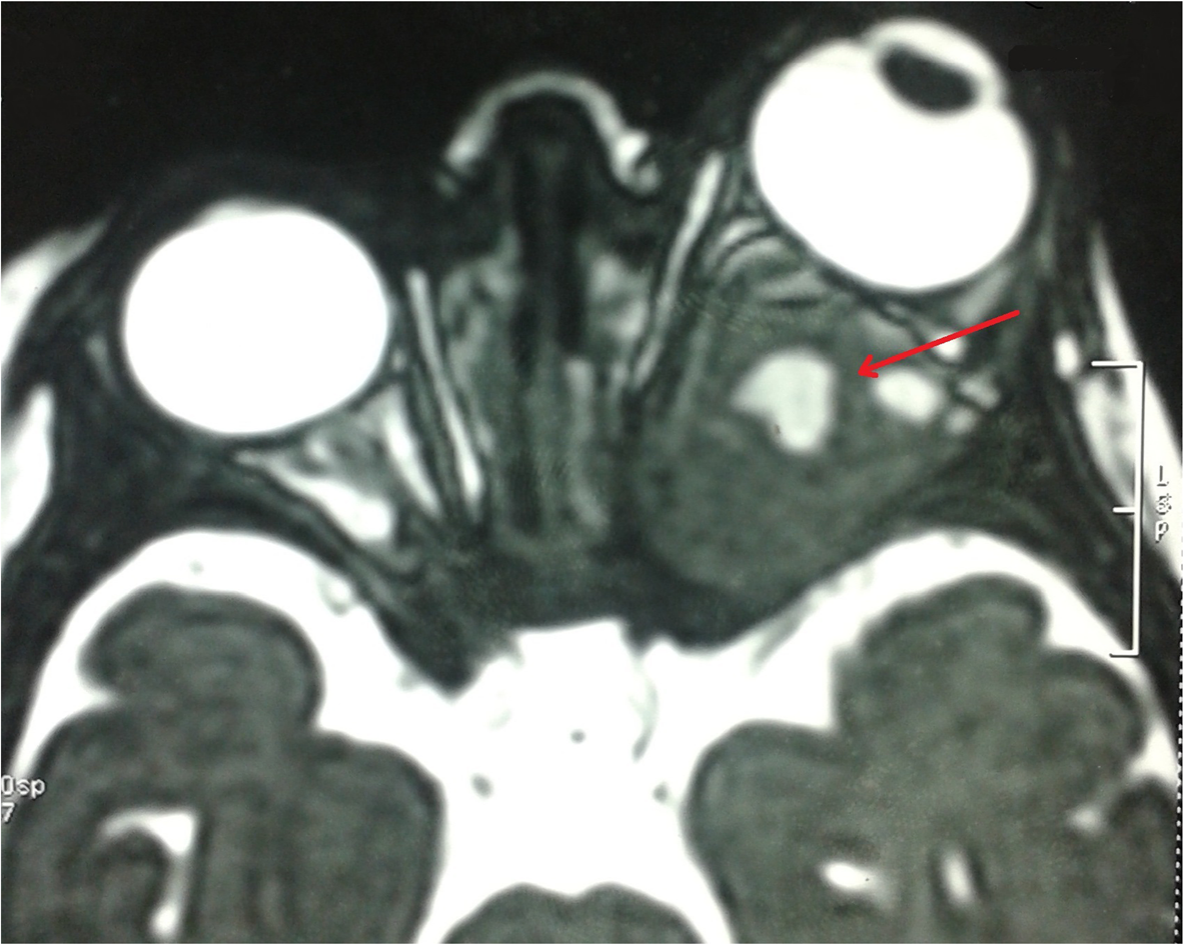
MRI aspect in admission. Axial T2-weighted MR image shows hyperintense signal and enlargement of the left optic nerve (arrow).
